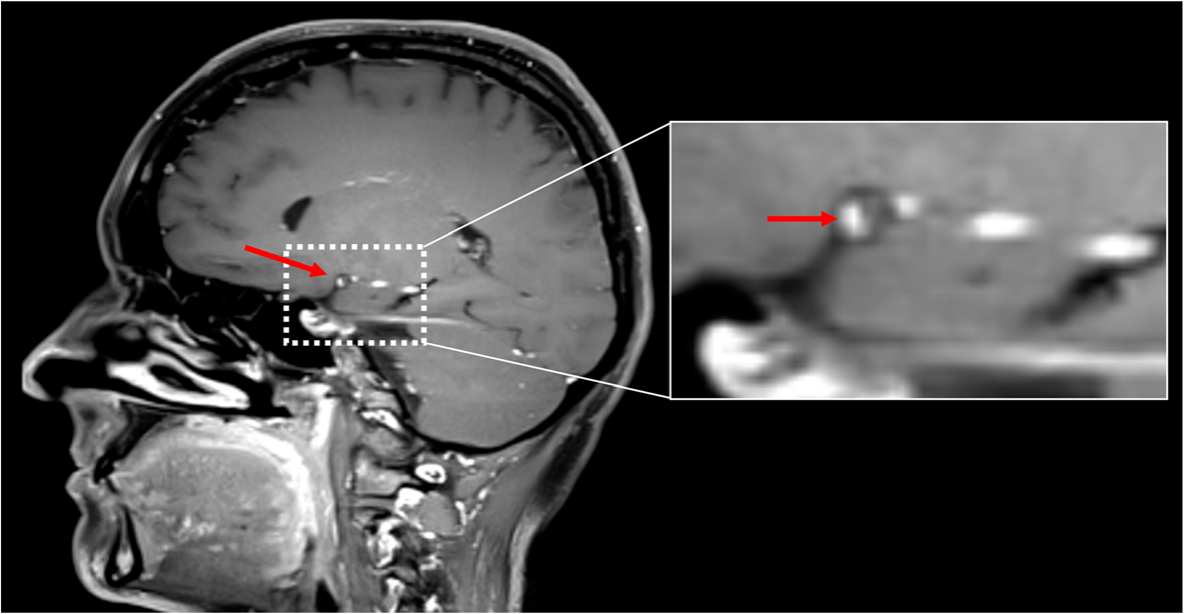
3-Tesla MRI of the brain. T1-weighted (volumetric T1 turbo spin echo) 3-Tesla MRI of the brain with fat suppression and gadolinium contrast showing focal vessel wall enhancement (arrow) of the right proximal middle cerebral artery
